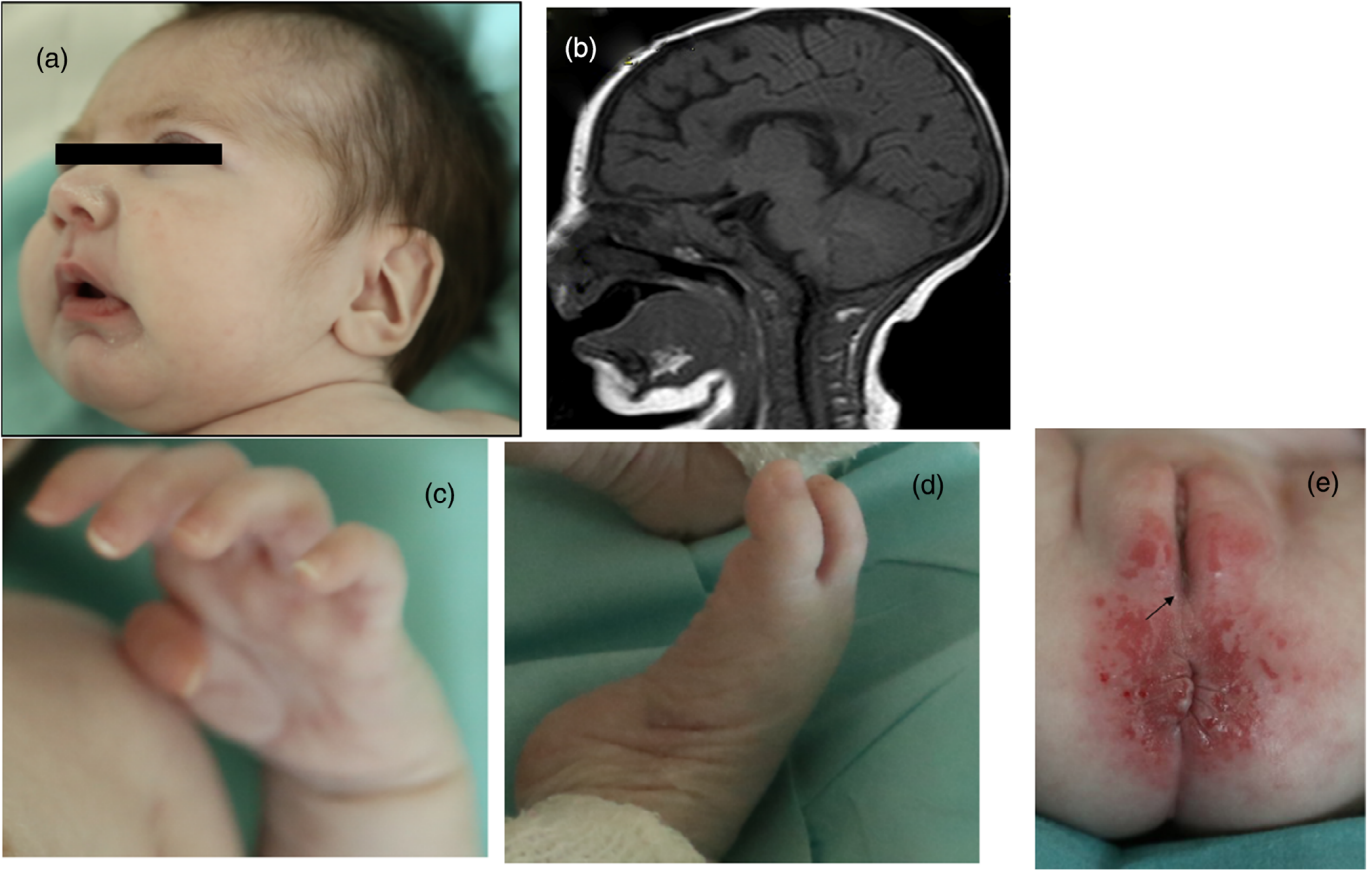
Patient with COG6‐CDG at the age of 3 months. (a) Facial dysmorphism (sloping forehead, thick nasal alae, mild retrognathia, large ears). (b) T1‐weighted sagittal brain magnetic resonance imaging shows partial agenesis of the corpus callosum and normal cerebellum. (c) distal arthrogryposis: opposed thumb, clenched fingers and (d) talipes equinovarus. (e) Normal anus and external genitalia, fecal discharges (not shown) per vaginam (arrow) were caused by congenital recto‐vaginal fistula
